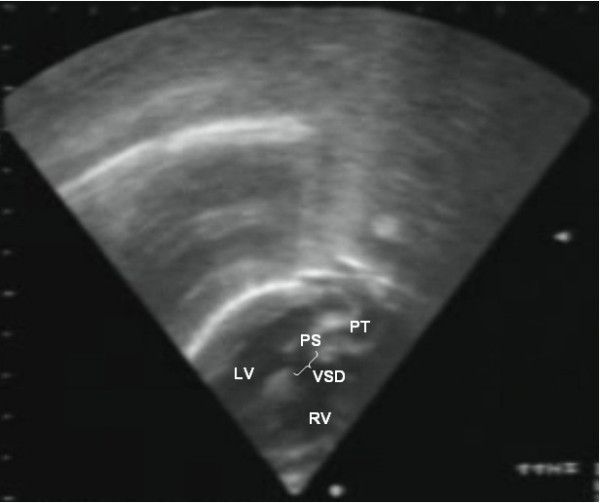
Echocardiography of a complex transposition with a ventricular septal defect and pulmonary stenosis.
